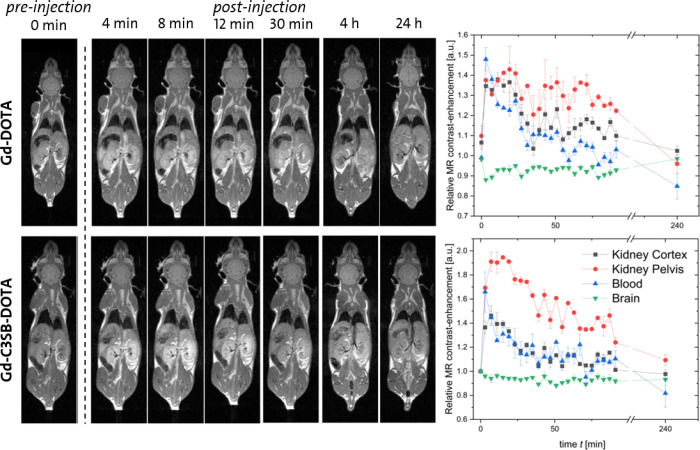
Dynamic contrast-enhanced MR images following
